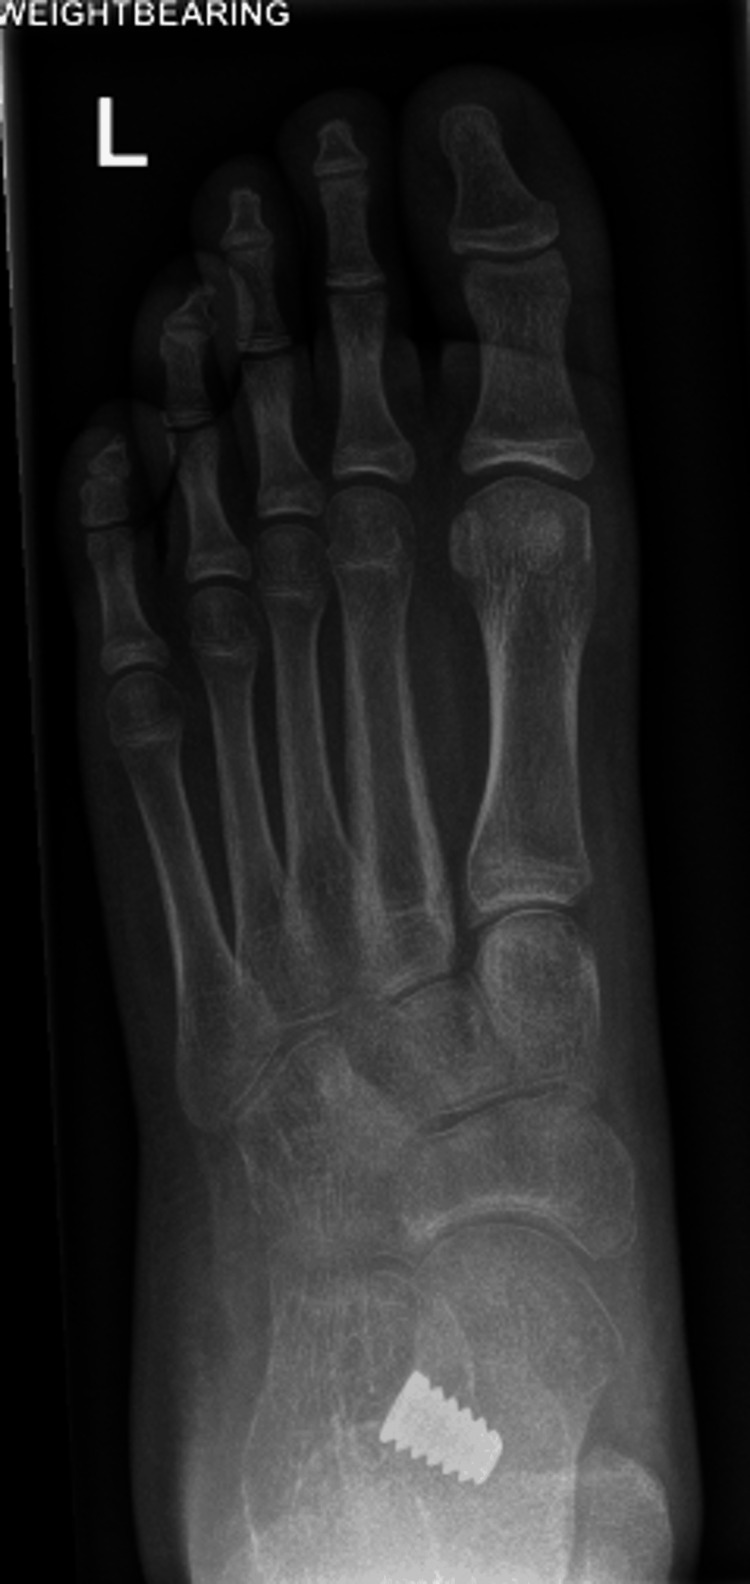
A post-operative weight-bearing DP radiograph of the left foot, demonstrating improvements in talonavicular uncoverage and Kite's angle DP: dorsoplantar
